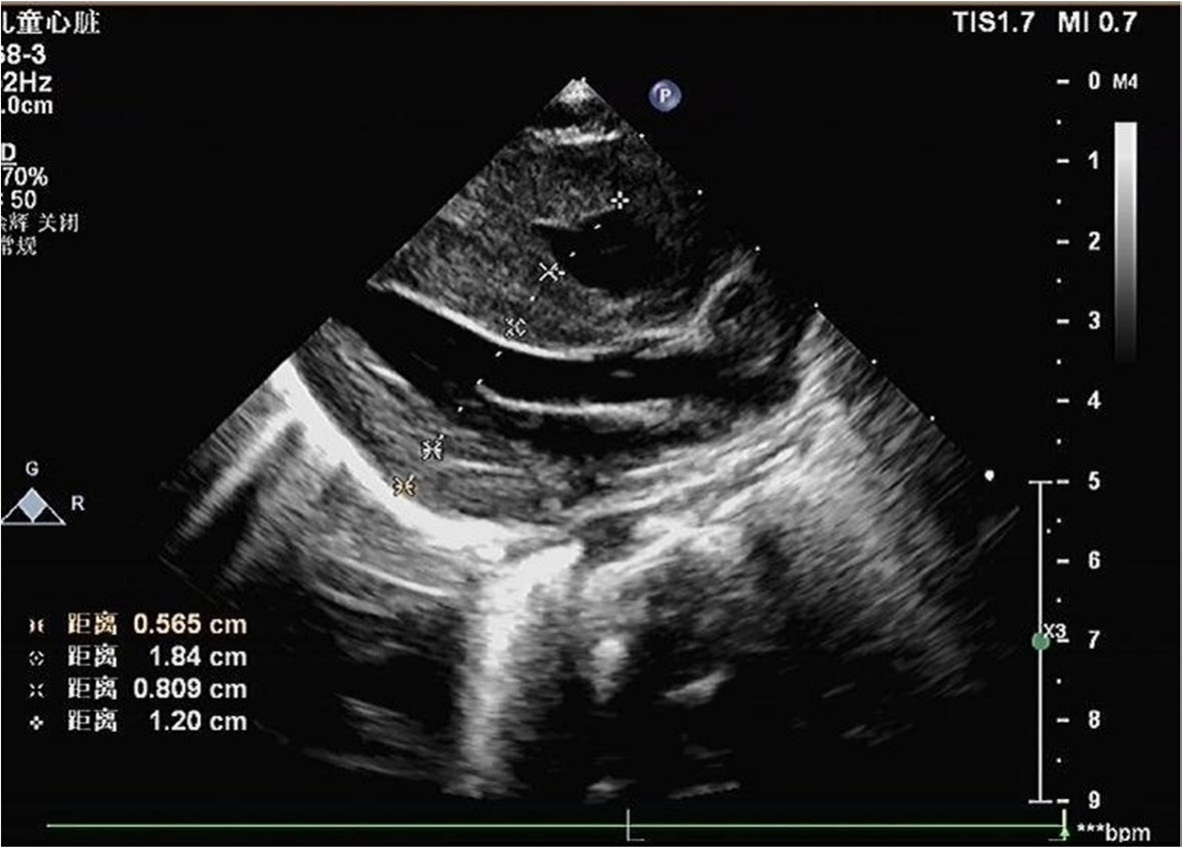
Echocardiogram demonstrating ventricular hypertrophy postnatally
